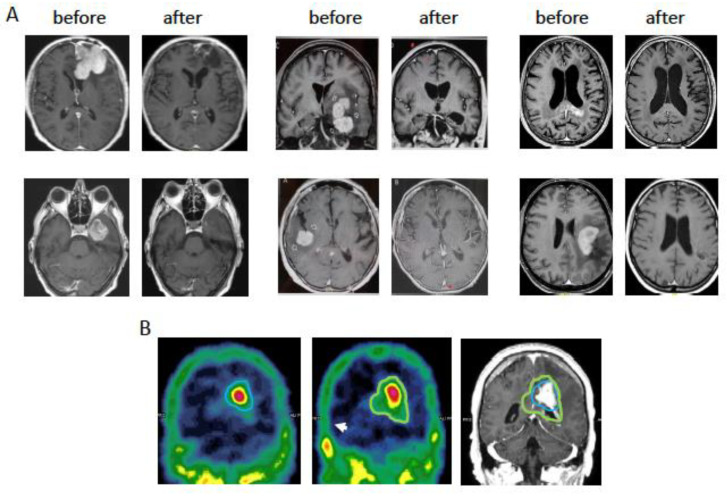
Examples of responses to NGR-hTNF/R-CHOP combination in patients with PCNSL. (A) DCE-MRI (gadolinium-enhanced T1-weighted scan) before and after treatment with 2–4 cycles of R-CHOP preceded by NGR-hTNF in 6 PCNSL patients. (B) SPECT analysis of a patient before and after the third treatment with NGR-hTNF and R-CHOP, showing an increase of uptake of 99mTc-DTPA. The volume of ≥30% uptake of 99mTc-DTPA is contoured in the two studies performed before (left image, blue line) and after (central image, green line) administration of NGR-hTNF/R-CHOP. The contoured volumes are also reported on the image obtained by gadolinium-enhanced T1-weighted MRI (right image) showing the tumor location. The volume of increased uptake before and after NGR-hTNF/R-CHOP administration was 22 and 40 cm^3^, respectively. Part of this research was originally published in *Blood* [24] and *Blood Advances* [23].
